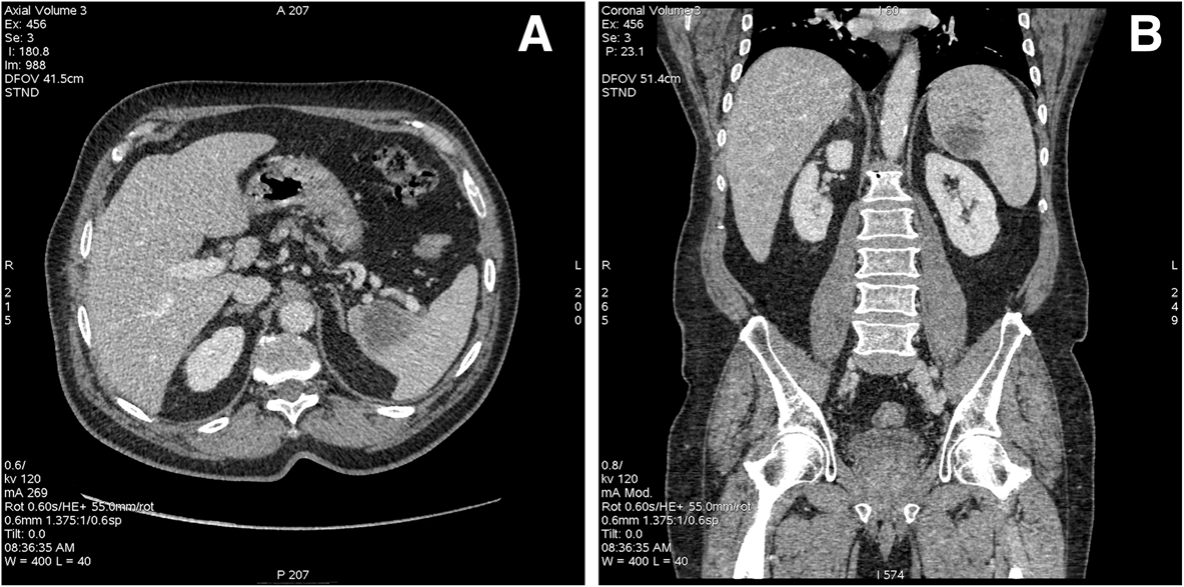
CT images of splenic metastasis. Axial section (A) and coronal section (B).
